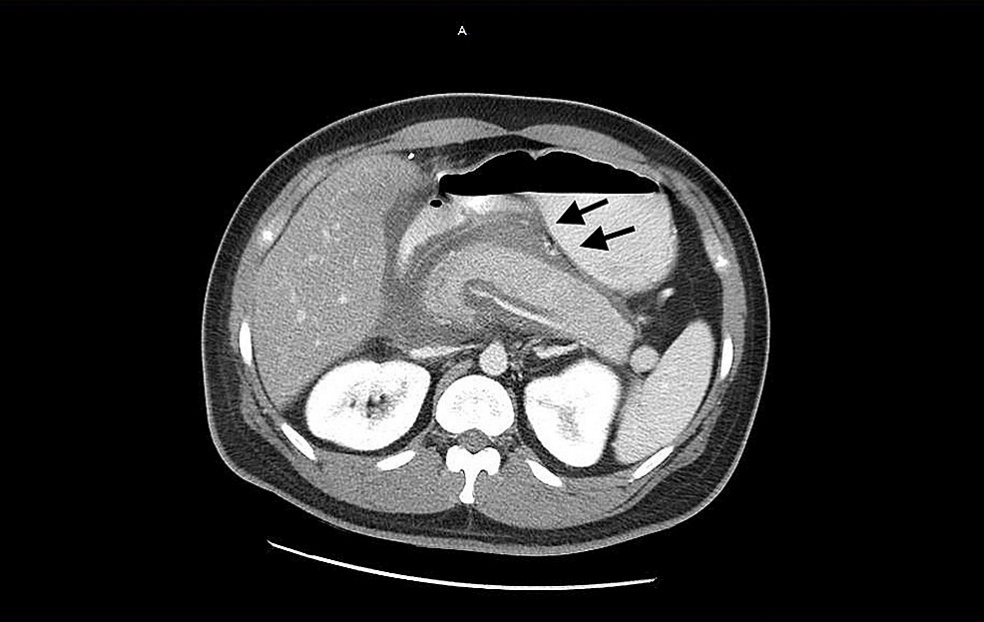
CT abdomen Imaging findings suggestive of severe acute pancreatitis with diffuse pancreatic enlargement, adjacent inflammatory changes, and free fluid.
